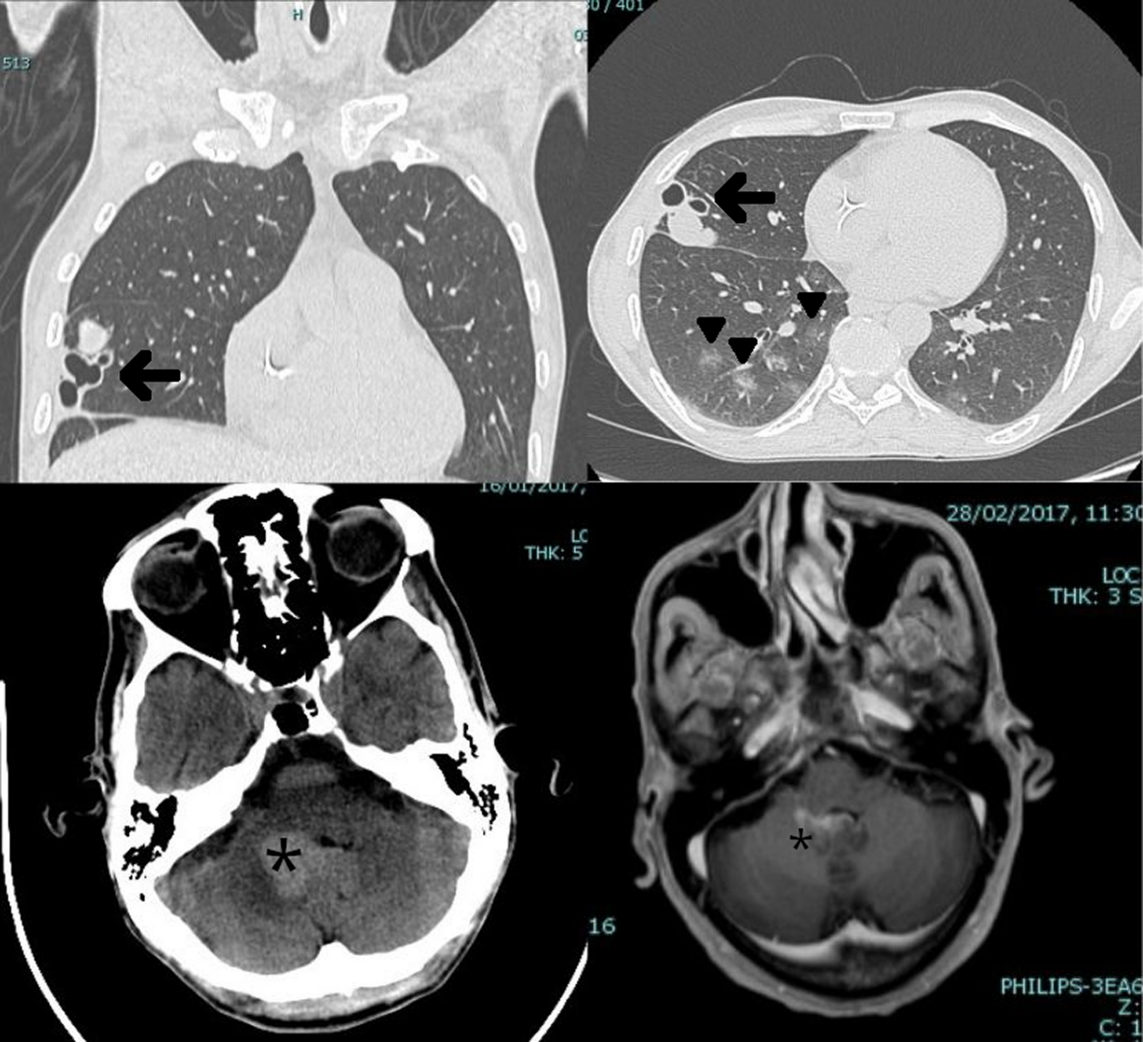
Top: coronal and axial chest CT scans demonstrating thick‐walled cysts (arrows) and ground‐glass opacities (arrowheads). Bottom: brain CT and MR imaging. The cerebellum was involved by an irregular lesion centered on the right tonsil and impinging on the fourth ventricle (asterisk)
